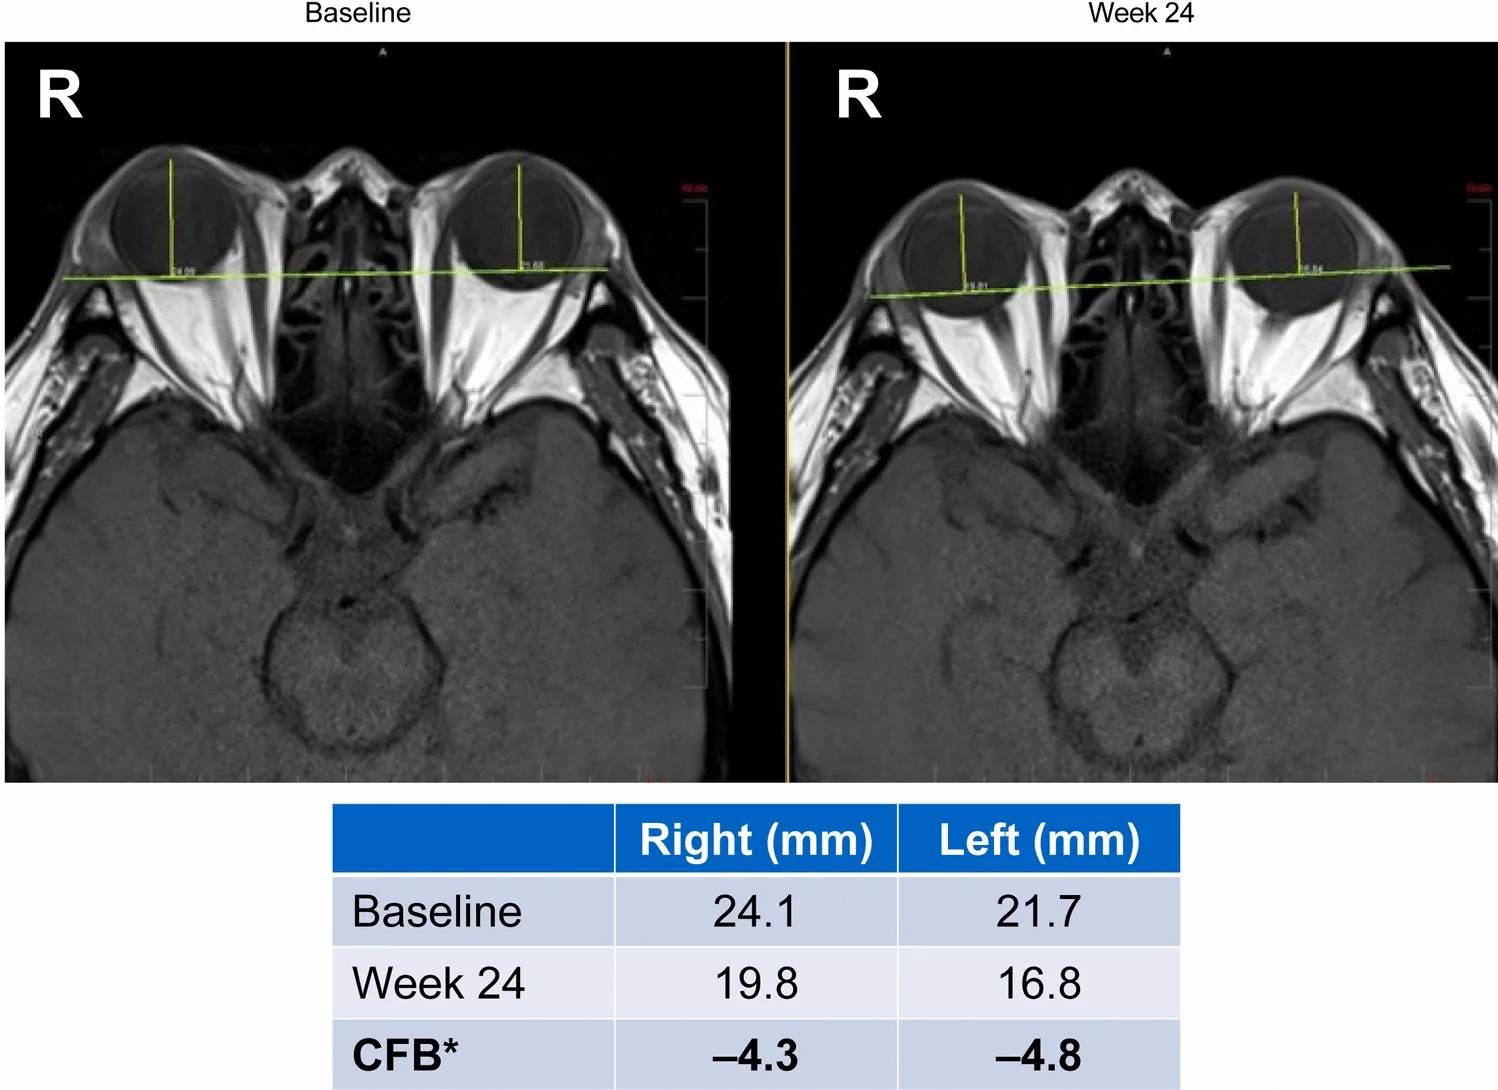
Confirmatory proptosis measurements of axial MRI scans for patient 4. *The CFB was calculated prior to rounding and may appear different than expected due to rounding. *CFB* change from baseline, *MRI* magnetic resonance imaging
